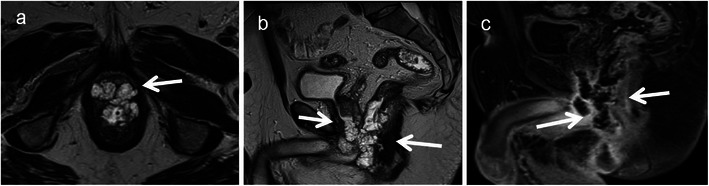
Mucinous (colloid) carcinoma of the rectum. a Axial and b sagittal T2-weighted MRI show intensely hyperintense heterogeneous rectal mass infiltrating the adjacent structures (T4 stage). (c) Sagittal fat-suppressed contrast-enhanced T1-weighted MRI reveal mesh-like peripheral enhancement of the rectal mass
